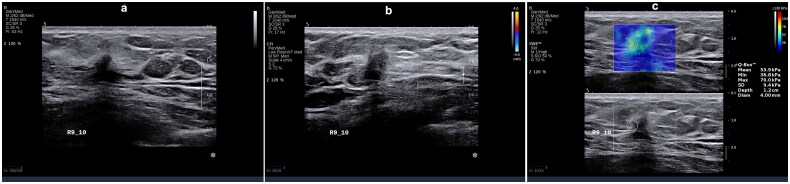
A 32-year-old female with right breast invasive ductal carcinoma grade II (score of 6), tumor size 1.0 × 0.8 × 0.5 cm, luminal a subtype. (a) Ultrasonography features: irregular shape, spiculated and angular margins, no change in posterior echo, no calcification. (b) Adler blood flow grading – grade 0 (c) Emax = 70.0 Kpa.
